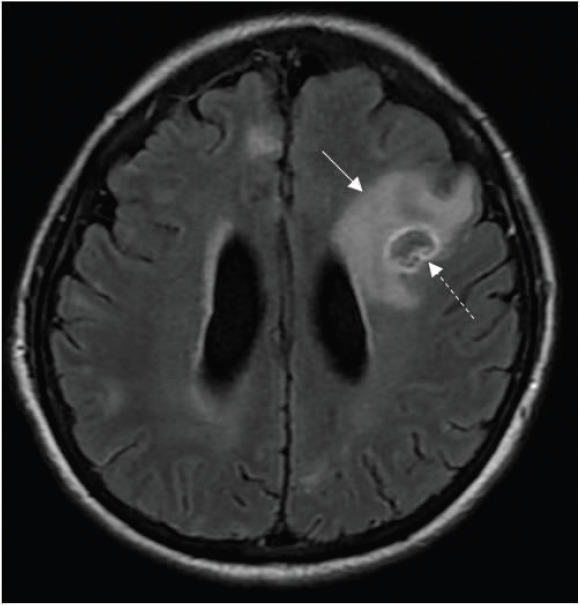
Axial T2-FLAIR post contrast-enhanced image showing a ring-enhancing lesion in the left frontal lobe with significant perilesional oedema (solid arrow). An eccentric focus of mural enhancement is seen (dashed arrow).
